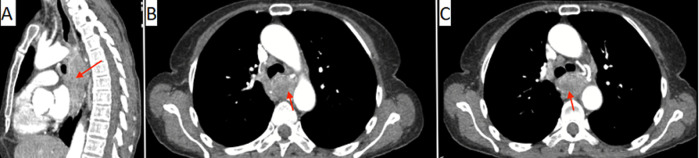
A,B,C) sagittal and axial images of the thoracic computed tomography scan with intravenous contrast showing the esophageal mass (red arrow) obstructing the lumen and presenting contact with the trachea and its two main bronchi
